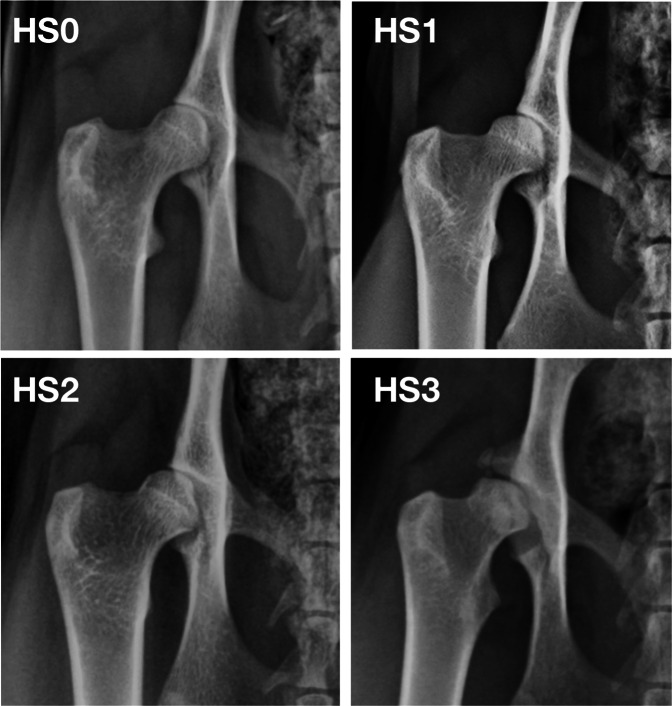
Examples of hip radiographs (right coxofemoral joint) taken from the PawPeds database showing examples of the range of possible hip score grades in the programme: (top left) hip score = 0 (HS0) normal hip with no evidence of FHD; (top right) hip score = 1 (HS1) hip with acetabulum covering < 50% of the femoral head; (bottom left) hip score = 2 (HS2) moderate radiographic signs associated with FHD including shallow acetabulum and deformation of the femoral head with some evidence of new bone formation around the joint; (bottom right) hip score = 3 (HS3) severe radiographic signs associated with FHD with very poor joint congruency, deformation of the femoral head and major changes associated with new bone formation around the joint.
